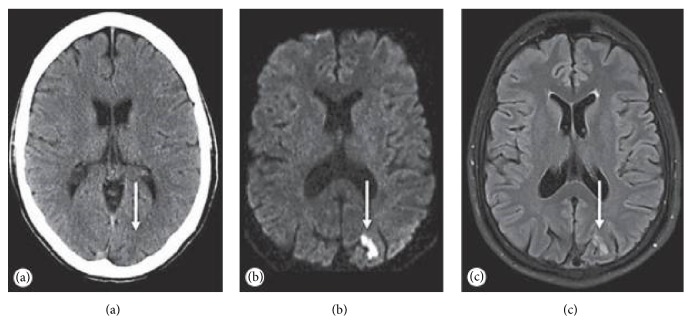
CT and MRI findings in a 49-year-old TIA patient. CT (a) diffusion-weighted (b) and T_2_-weighted FLAIR MRI (c). (b and c) Show a small acute ischemic lesion (arrow) in the left posterior cerebral artery territory. However, CT is not clearly [20].
